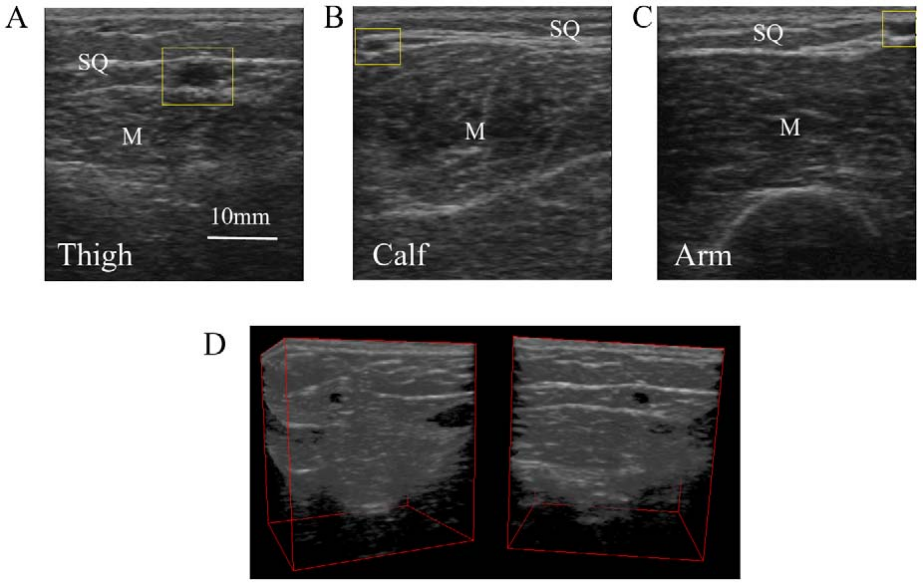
Ultrasound images showing the subcutaneous blood vessels encapsulated by fascial bands. (A). From the site of thigh; (B). From the site of calf ; (C) From the site of arm; (D). Three-dimensional reconstruction of a site with a vessel.
